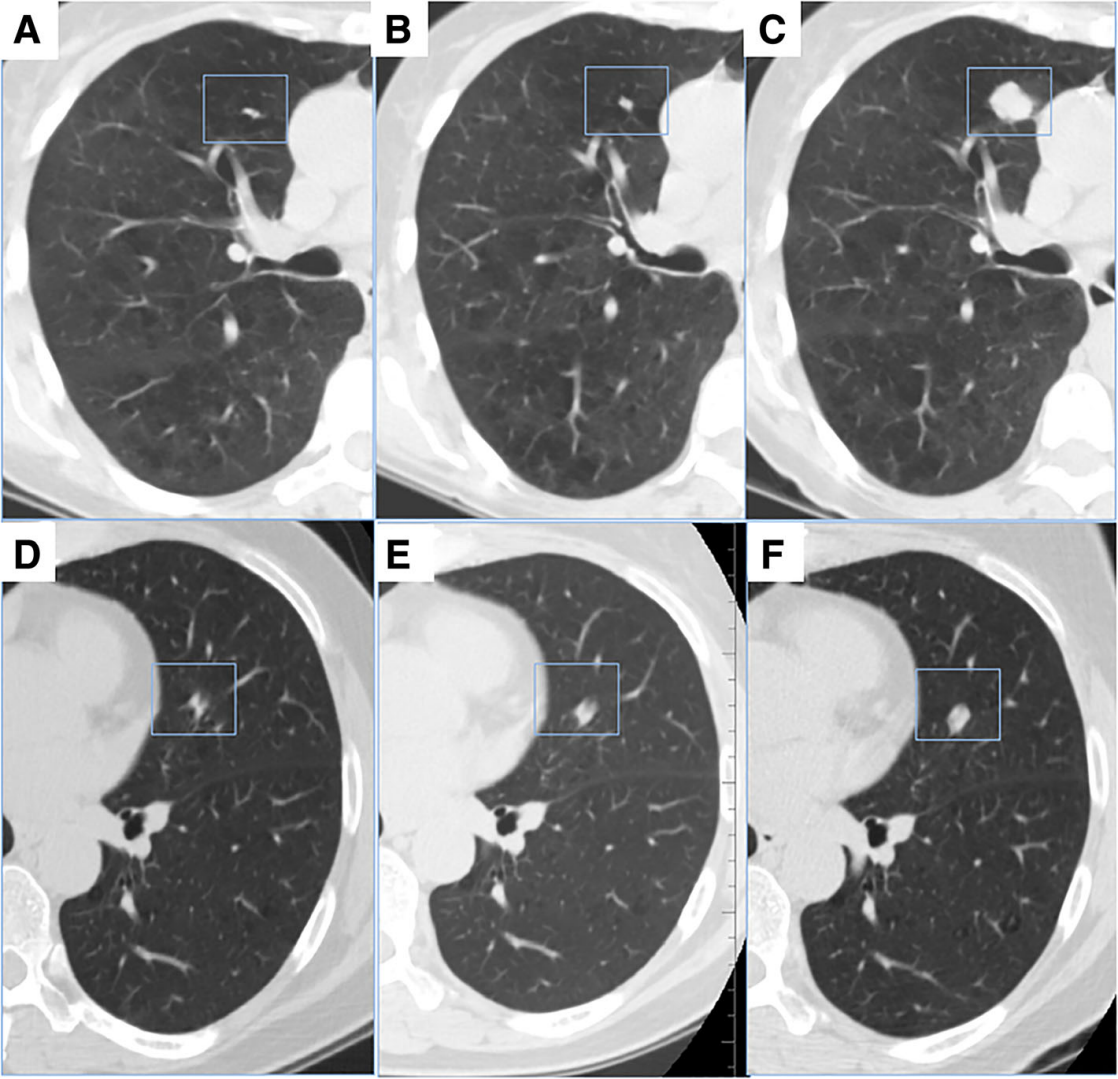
The lung cancers with mixed growth pattern during two round of follow up scan. a-c A nodule re-classified from indolent to aggressive. a Baseline scan (T0). Axial CT images show an irregular nodule in right upper lobe. b The first follow up (T1), with the interval days of 406 days and VDT 5713 days. c The second follow up (T2), with the interval days of 355 days and VDT 86 days. d-f A nodule re-classified from aggressive to indolent cancer. d Baseline scan (T0). Axial CT images show an amorphous nodule in left upper lobe. e The first follow up (T1), with the interval days of 430 days and VDT 114 days. f The second follow up (T2), with the interval days of 300 days and VDT 848 days
